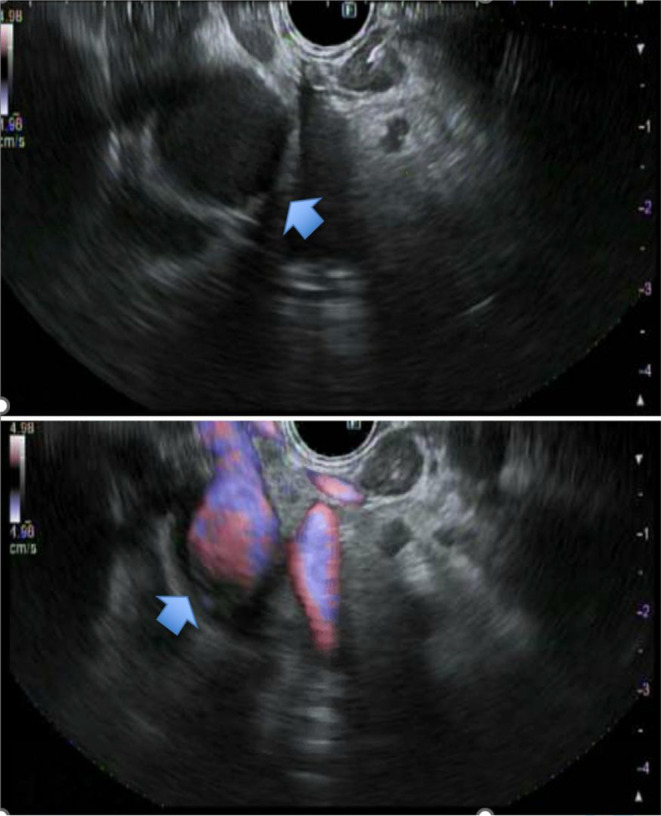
(A and B) shows an EUS with prominent lymph nodes in the perigastric and periduodenal region. (A) Shows the largest lymph node measuring 15 mm in maximal cross‐sectional diameter. (B) Shows doppler of the previously mentioned lymph nodes.
